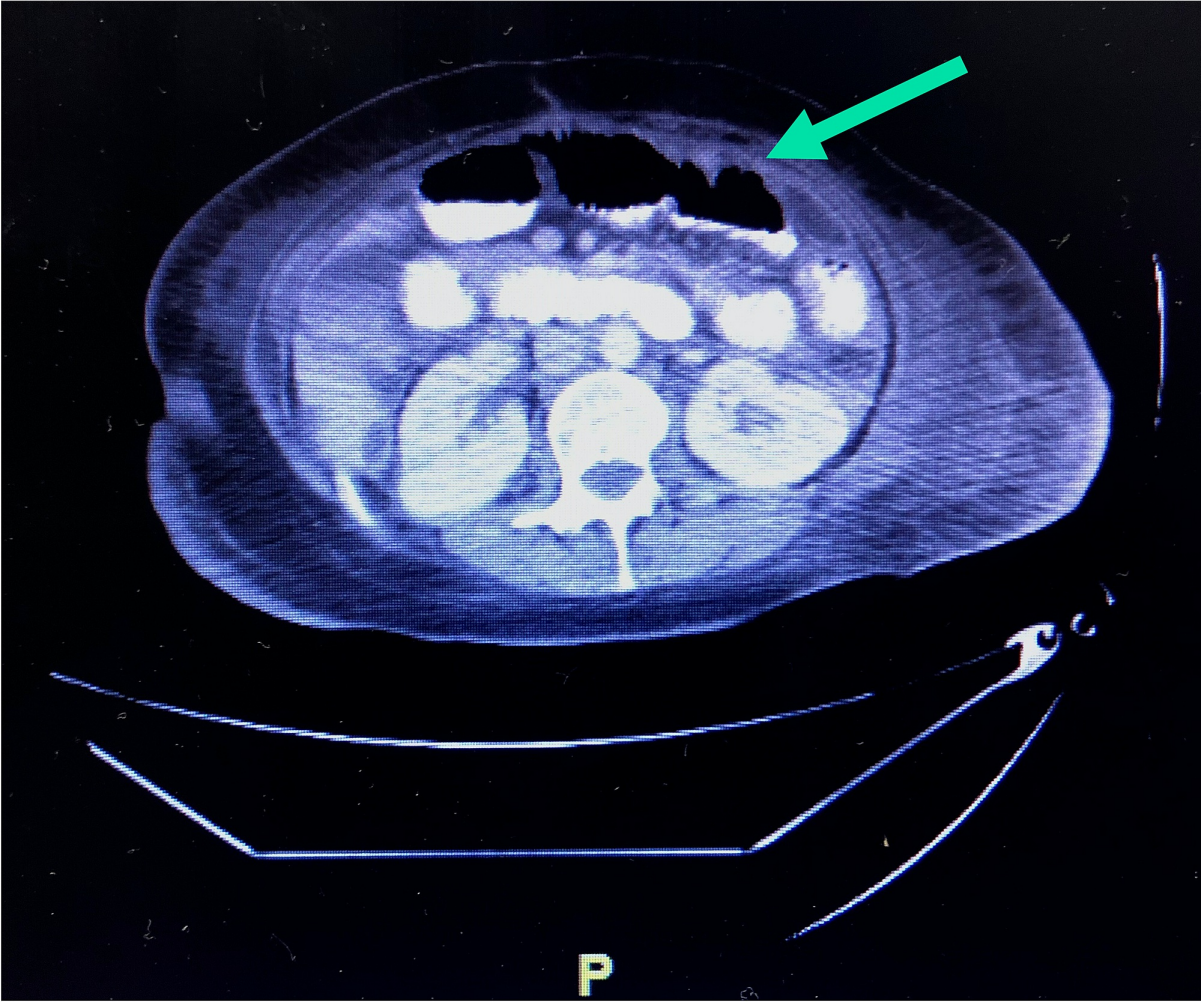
Abdominal computed tomography scan demonstrating diffuse dilation of the small bowel with a focal transition point in the lower quadrant of the abdomen, findings compatible for a worsening high-grade distal small bowel obstruction. Bowel contrast was noted within the proximal and mid small bowel with mild mucosal thickening and decompressed colon.
